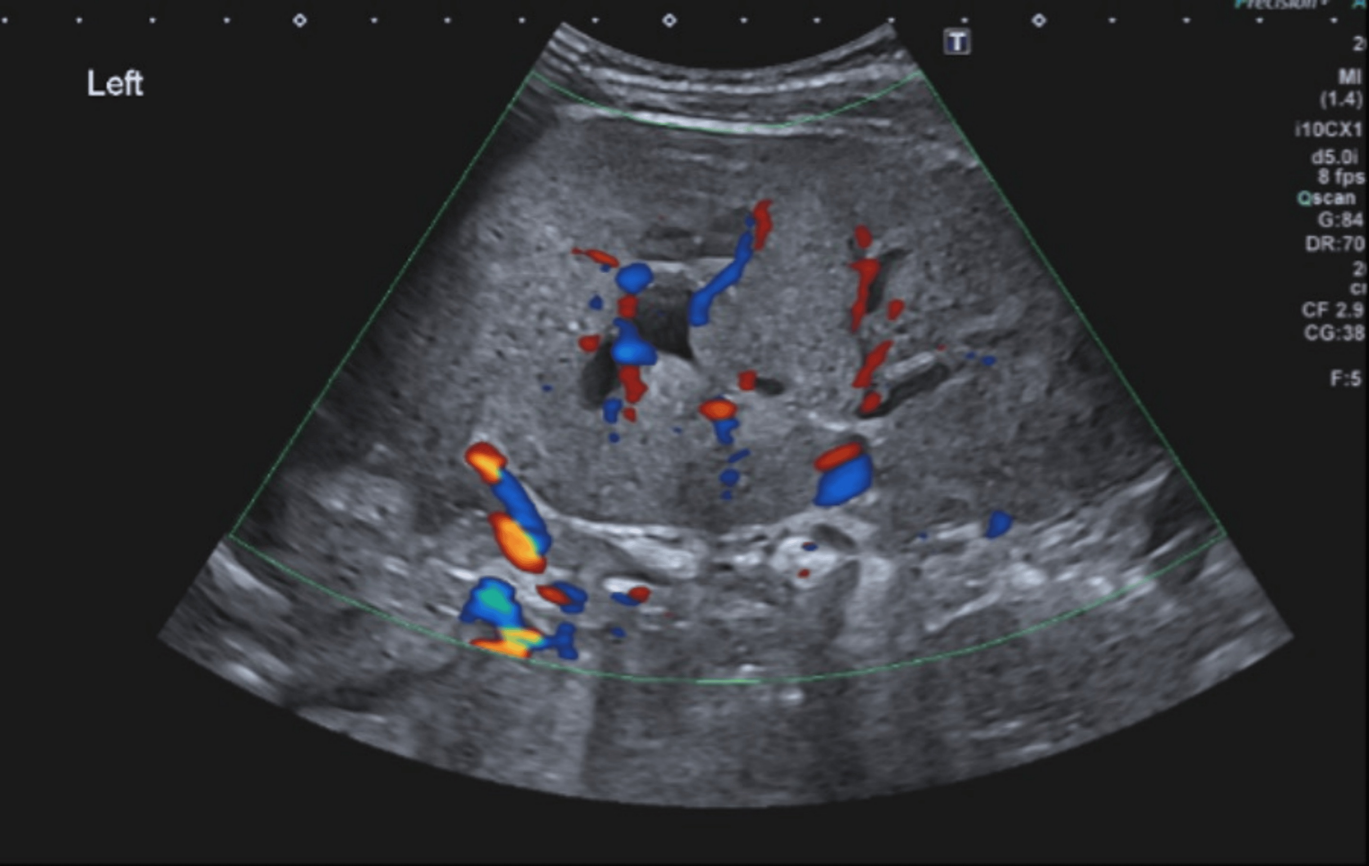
Abdominal Doppler ultrasonography of left kidney demonstrating no abnormality of the vascularity of the kidney
